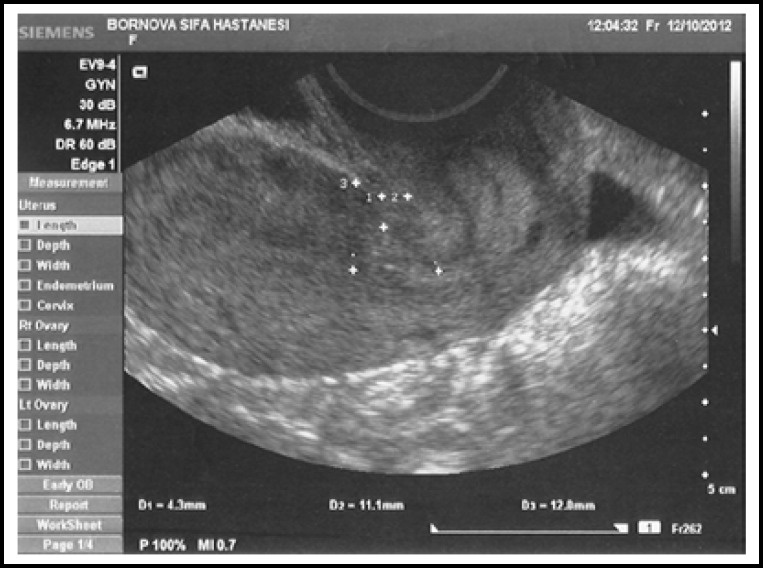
Ultrasound image of a uterus with locked method.
